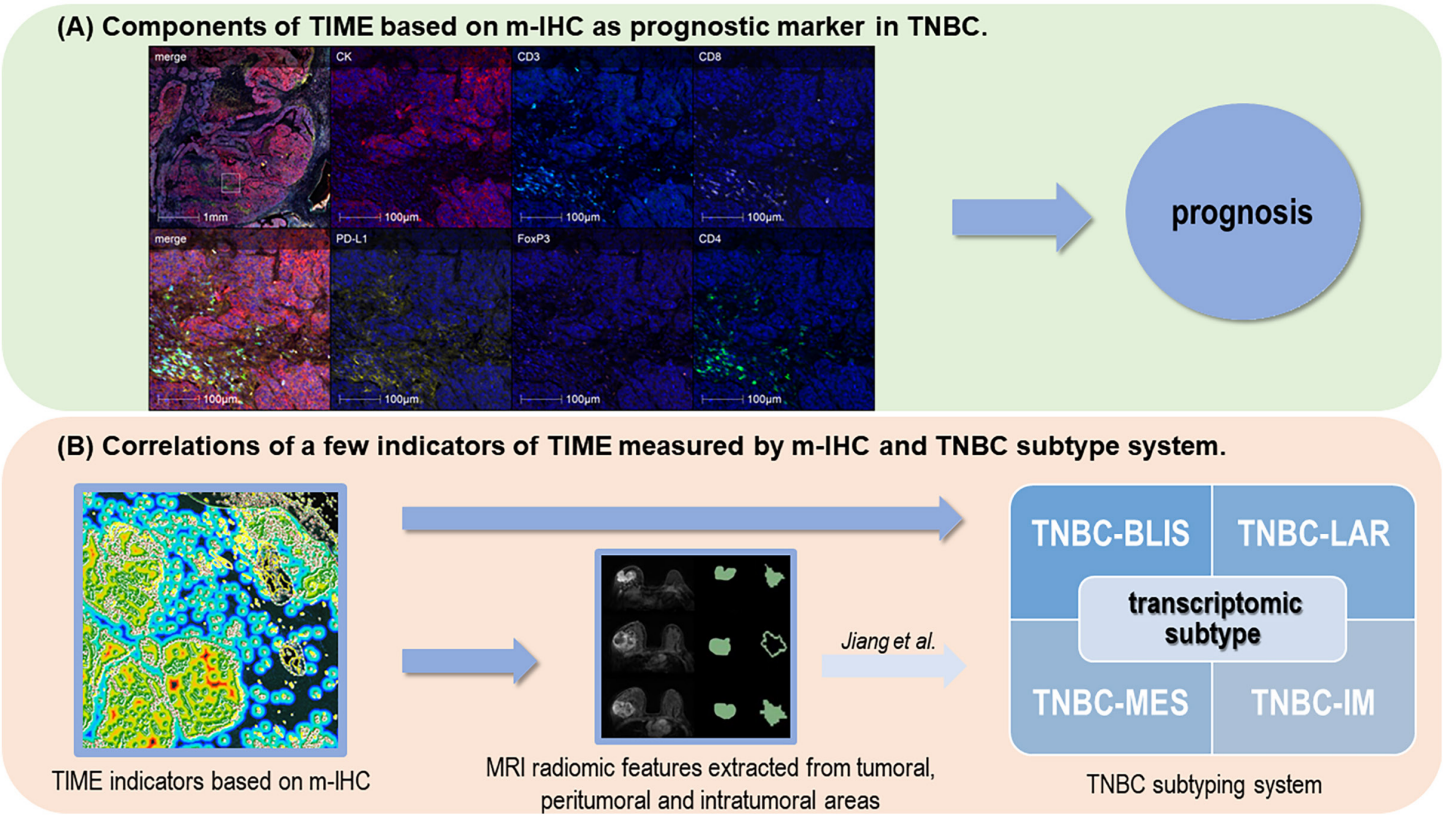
Overview of this research. (A) Components of the tumor immune microenvironment (TIME) based on m‐IHC were found to be effective predictive markers of prognosis in TNBC patients. Markers of m‐IHC were Pan‐CK, CD8, PDL1, CD3, CD4, and FoxP3. Prognosis included overall survival (OS), relapse‐free survival (RFS), and distant metastasis‐free survival (DMFS). (B) A few indicators of TIME measured by m‐IHC could complement the TNBC subtyping system. The TIME indicators correlated with TNBC transcriptomic subtypes, namely, the TNBC‐luminal androgen receptor (TNBC‐LAR), TNBC‐immunomodulatory (TNBC‐IM), TNBC‐basal‐like immune‐suppressed (TNBC‐BLIS), and TNBC‐mesenchymal‐like (TNBC‐MES) subtypes. The TIME indicators also correlated with MRI radiomic features reflecting TNBC subtypes.^15^
